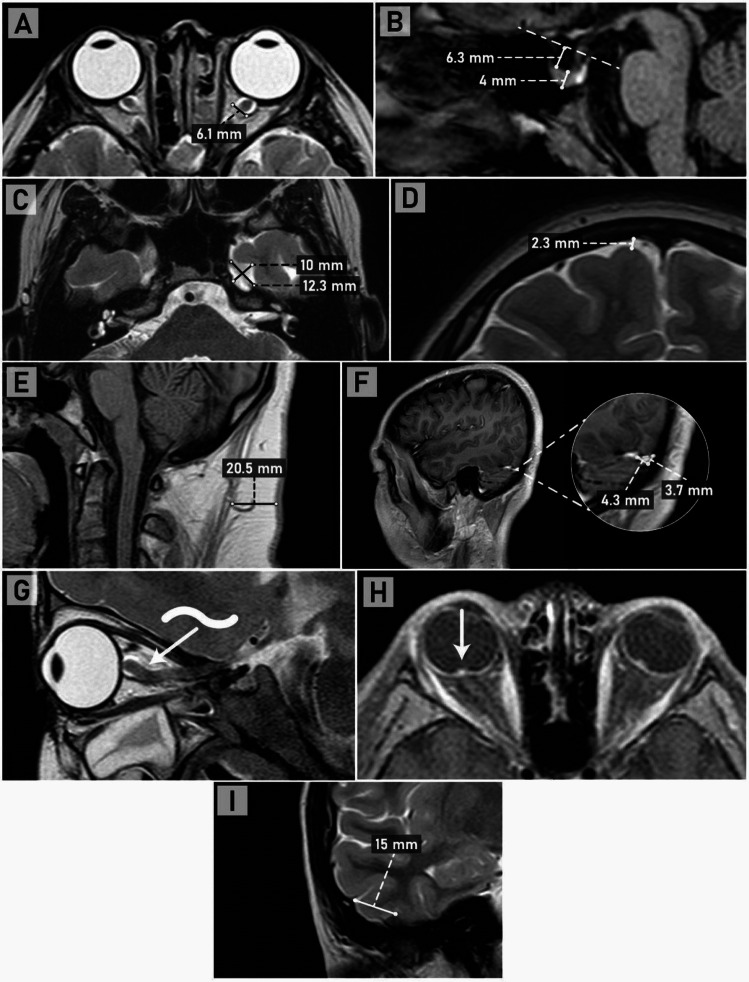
Interpretation of the MRI signs of ICH. A Bilateral increase in the subarachnoid space around the ON in axial T2 and flattening of the posterior sclera. B Sagittal T1 showing an “empty” (CSF occupied) *sella turcica* greater than 50%. C Axial T2 with increased amplitude of Meckel’s cave. D Subarachnoid space of the convexity. E Nuchal fat fold thickening, measured in sagittal T1. F Post-contrast 3D sagittal T1 image showing the measurement of two diameters of the left transverse sinus, triangular in shape, when comparing with a reference point 1.5 cm away from the torcula within the same sinus (not shown); there is a difference greater than 50% of the area, confirming stenosis. G Parasagittal T2 section of the orbit showing vertical tortuosity of the optic nerve. H Bilateral flattening of the posterior sclera, enhancement, and intraocular protrusion of the optic nerve head on axial contrast-enhanced T1 with fat saturation (arrow). I Positive temporal “thumb” sign on coronal T1
